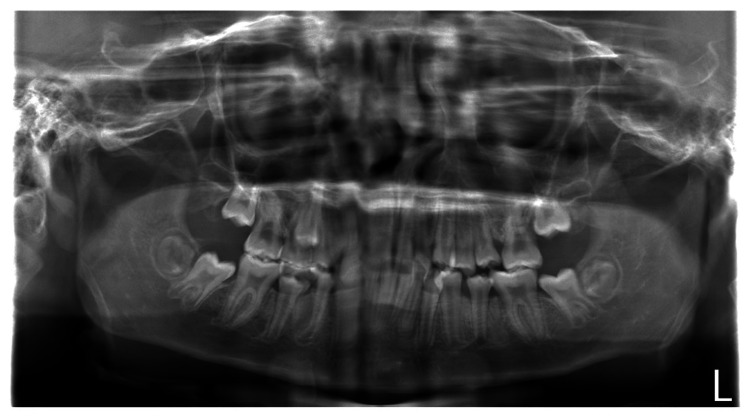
Panoramic radiograph of older brother with SDS SDS: Shwachman–Diamond Syndrome
